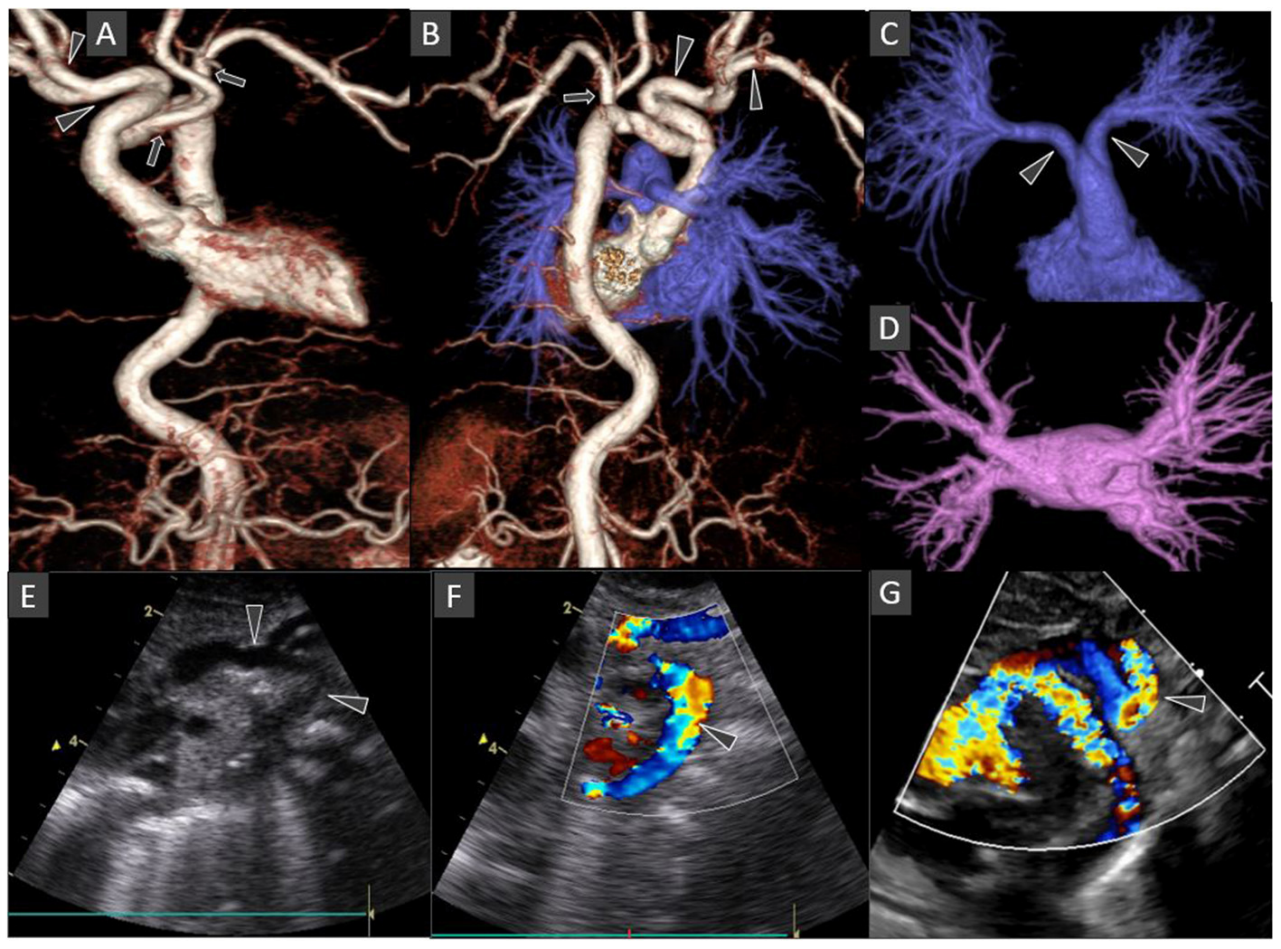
Chest CT Angiography 3D surface shaded display images: Anterior view of the thoraco-abdominal aorta (A); Posterior view of the combined thoraco-abdominal aorta and pulmonary arteries (B); Antero-superior view of the subtracted pulmonary arterial circulation and right atrium (C); Superior view of the subtracted pulmonary venous circulation and left atrium (D). Oblique coronal gray-scale (E); and color Doppler (F,G) echocardiography images of the aortic arch and its major branches. The left-sided aortic arch has an unusual configuration with the dilated innominate artery forming a hairpin turn before its bifurcation into right subclavian and common carotid arteries (arrowheads in A,B). The left common carotid and subclavian arteries arise from the hypoplastic mid arch (arrows in A,B). The thoraco-abdominal aorta is tortuous. Included abdominal aorta shows dilated and tortuous branches of the coeliac axis. The main pulmonary artery is short with early bifurcation with long tubular configuration and parallel proximal course of the right and left main pulmonary arteries (arrowheads in C). Normal morphology of bilateral pulmonary veins draining into the left atrium (D). Some of these findings are also demonstrated on echocardiography images (arrows in E–G).
